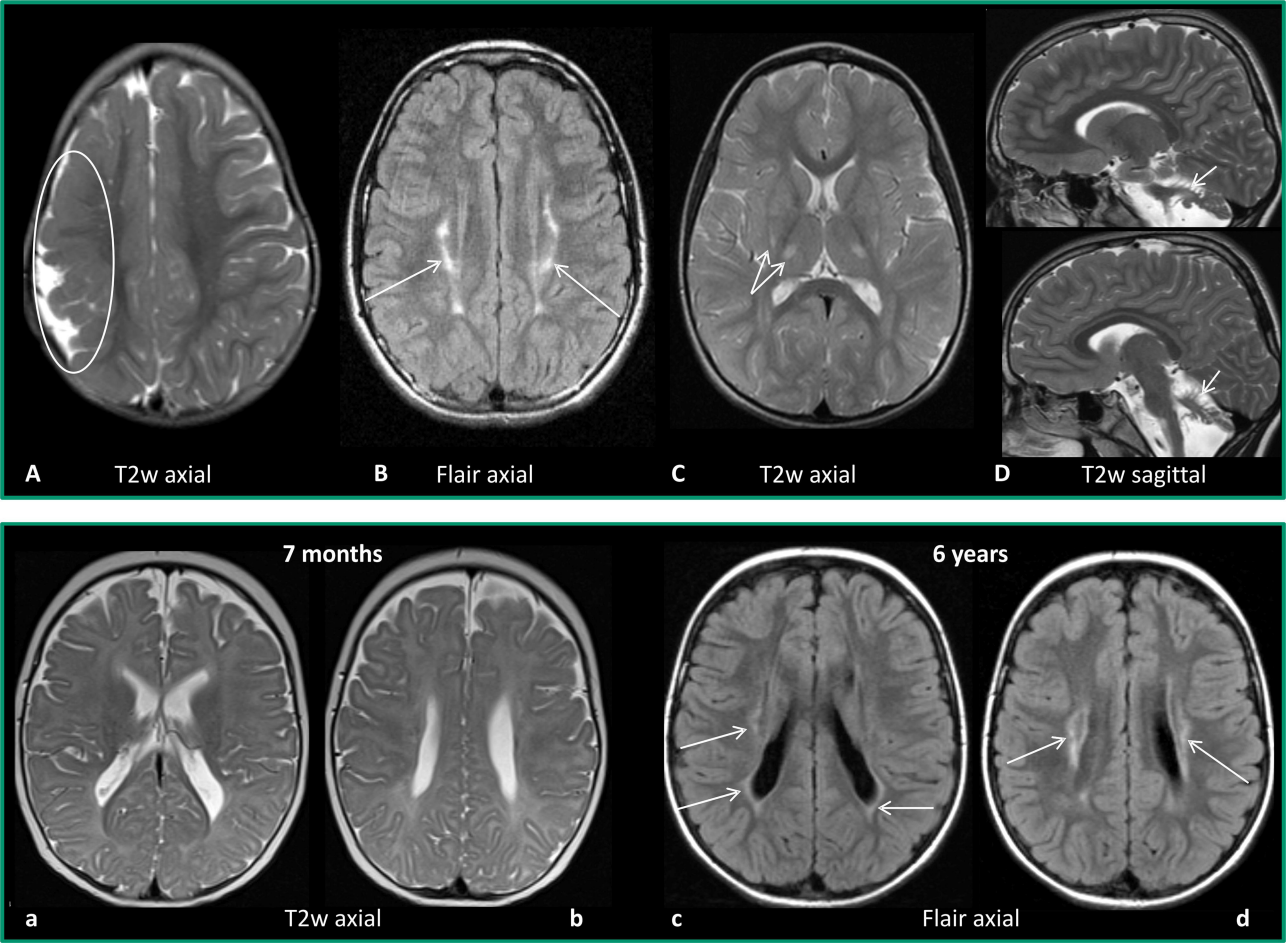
Upper row: Examples for MRI patterns. (A) Maldevelopment: frontoparietal polymicrogyria right (encircled), 2 years old girl with US-CP left GMFCS I. (B) Predominant white matter injury: mild PVL (arrows), 12-year-old girl, preterm 32 weeks of gestation, BS-CP GMFCS I. (C) Predominant gray matter injury: mild basalganglia/thalamus lesions (double arrow), 3-year-old girl, placental ablation, dyskinetic CP GMFCS II. (D) Miscellaneous: cerebellar atrophy (arrows), 2-year-old boy, former preterm 26 weeks of gestation, ataxic CP, GMFCS I. Lower row: Example for difficulty of early diagnosis–PVL, former preterm, 32 weeks of gestation. (a,b) MRI at the age of 7 months: T2w axial images with no clear pathology. (c,d) MRI at the age of 6 years (BSCP, GMFCS III): axial FLAIR images reveal bilateral gliosis indicating PVL (arrows).
